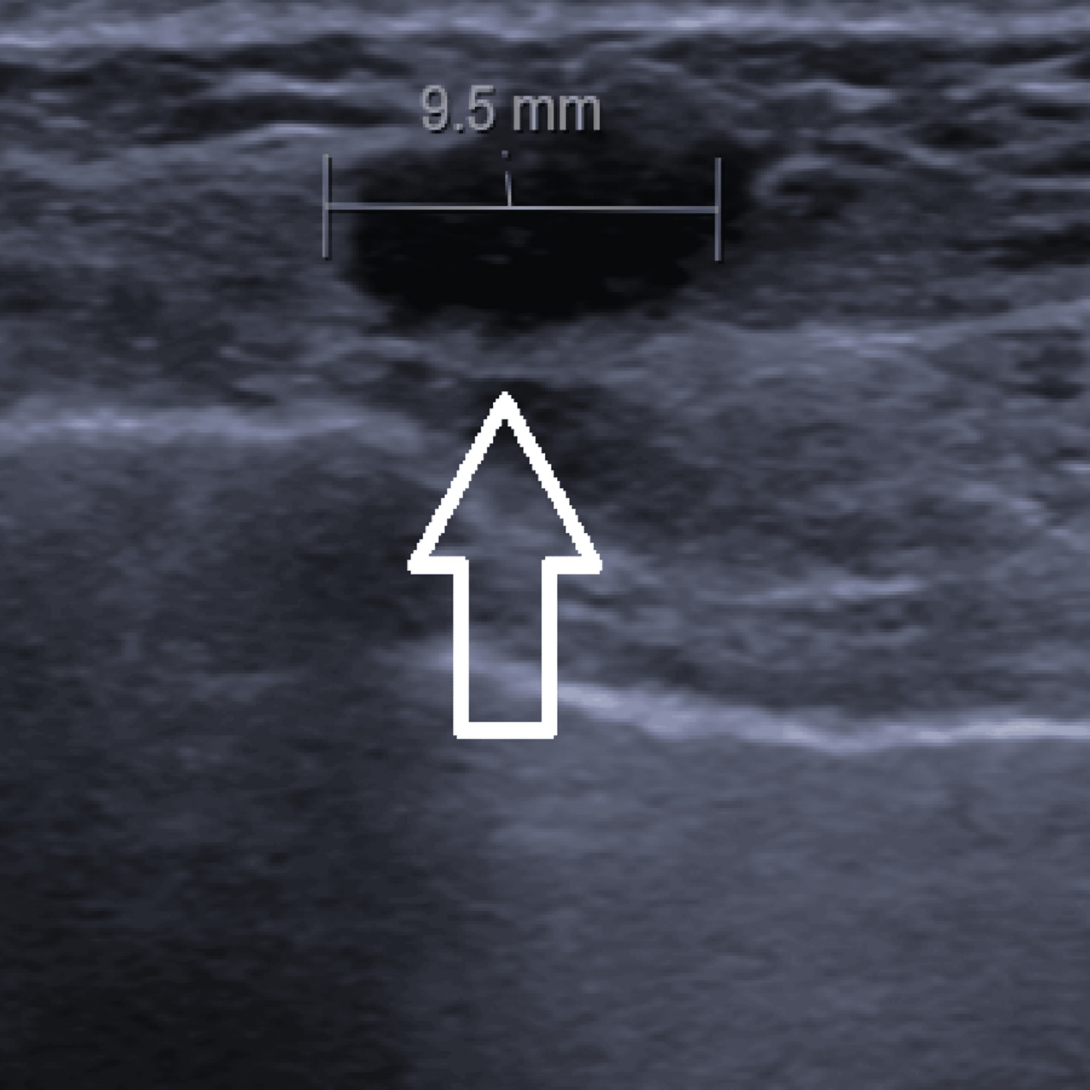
Ultrasound of breast demonstrating 9.5 mm nodule at the edge of left mastectomy scar (white arrow).
